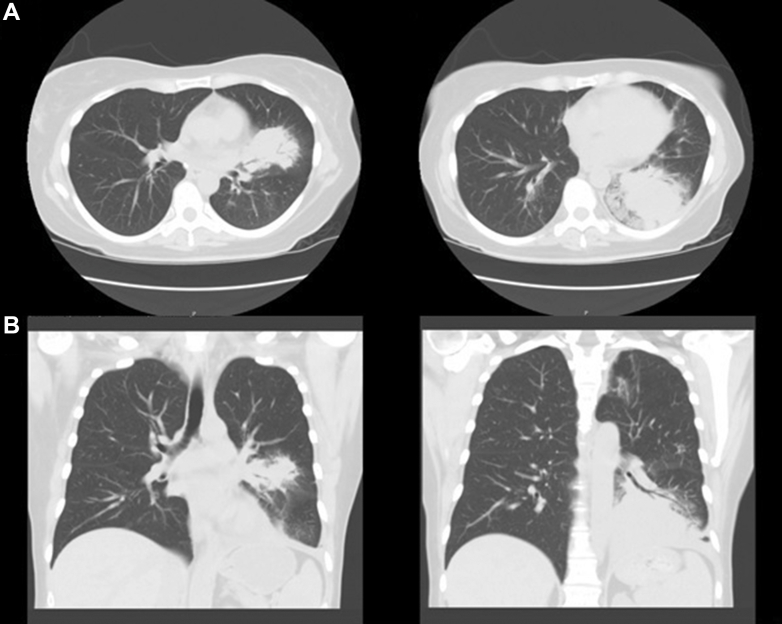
Axial (A) and coronal (B) CT imaging demonstrating ill-defined mass-like consolidations in the left upper and lower lobes.
